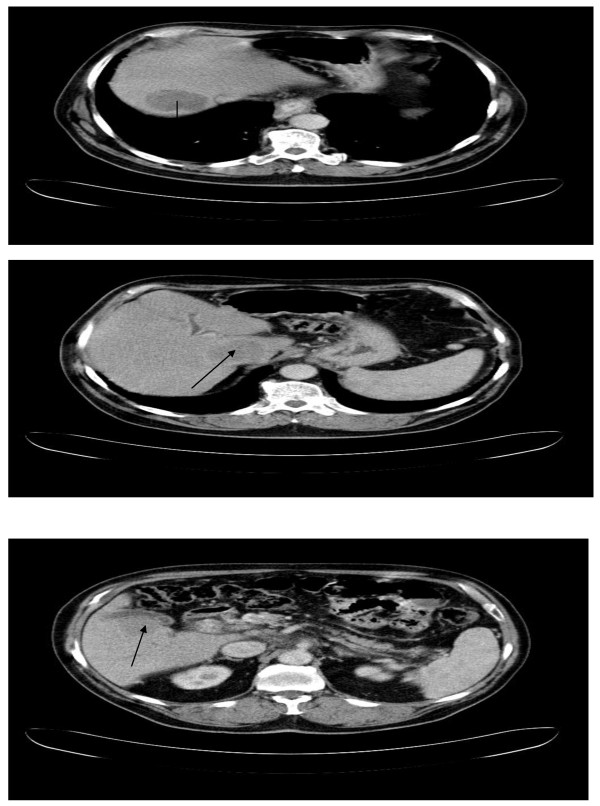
(A-C) CT scans (May 2005): HCC lesions in segment 7, caudate lobe, segment 5 of liver, respectively.
